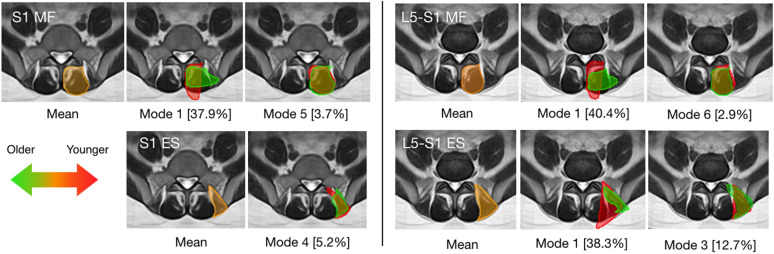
Modes of the multifidus (MF) and erector spinae (ES) shape that were associated with age. The mean shape is shown in orange overlaid on population-averaged MRI templates. The trend of shape variation towards an older age is in green and the opposite is in red. For each mode, threefold standard deviations from the mean shape are depicted for both directions of shape variants, and the percentages of shape variance explained are included in [ ] for all modes.
